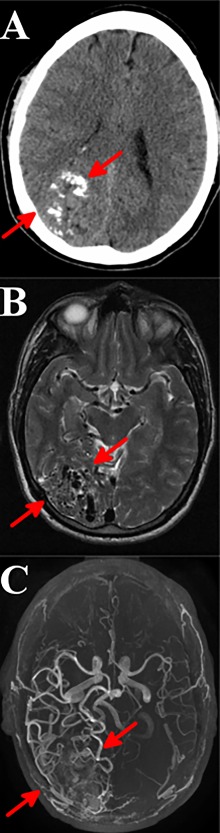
Computerized tomography (CT), MRI and angiography (MRA) axial images of a patient diagnosed with a BAVM. The initial diagnosis of BAVM is based on behavioral symptoms and on differentiation from other brain disorders. Multiple structural imaging techniques are often required to definitively diagnose the arteriovenous syndrome. Image (A; red arrows) depicts an unenhanced axial CT image at the level of the lateral ventricles demonstrating a cluster of serpiginous coarse calcifications in the right occipital lobe which is suggestive of vascular calcification. Image (B; red arrows) depicts an axial T2‐weighed MR image at the level of the midbrain demonstrating several prominent flow‐voids in the right occipital lobe which is suggestive of an arteriovenous malformation. Image (C; red arrows) depicts an unenhanced reconstructed axial time‐of‐flight maximum‐intensity projection image of the Circle of Willis demonstrating a right posterior arteriovenous malformation with feeding arteries from the right posterior cerebral artery and branches of the right middle cerebral artery.
